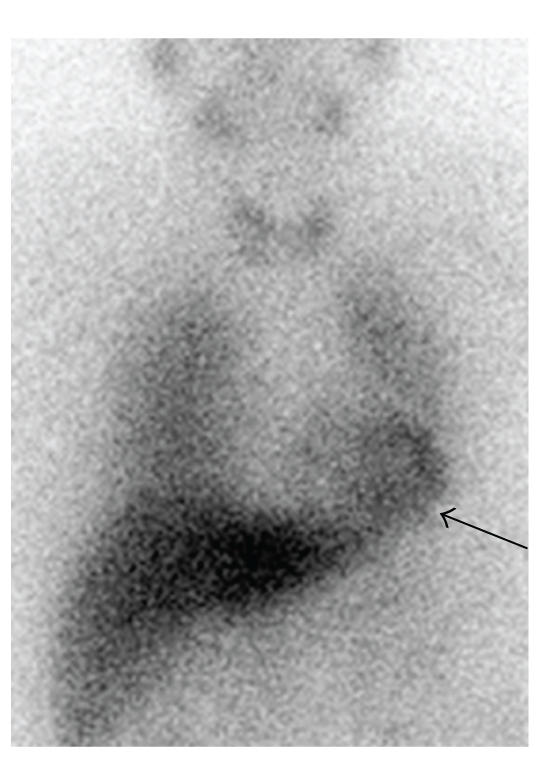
MIBG scintigraphy in a healthy volunteer. Anterior-posterior view in a 64-year-old healthy volunteer. The measurement was performed four hours after intravenous MIBG application. There is an intensive myocardial MIBG uptake (black arrow).
